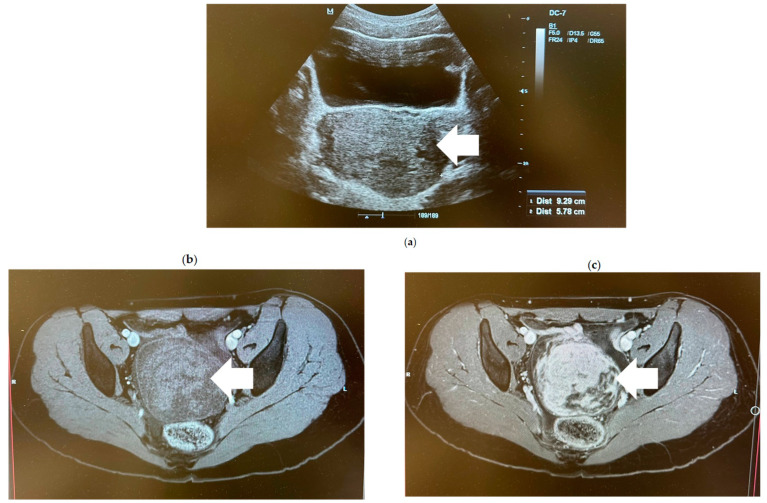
Radiologic evaluation. (a) Transabdominal ultrasound image showing a solid mass (arrow) in the right ovary. (b,c) Axial pelvic MRI images showing the right ovarian heterogenic structure of a solid mass (arrow) with a capsule that enhanced intensely after the administration of contrast medium.
